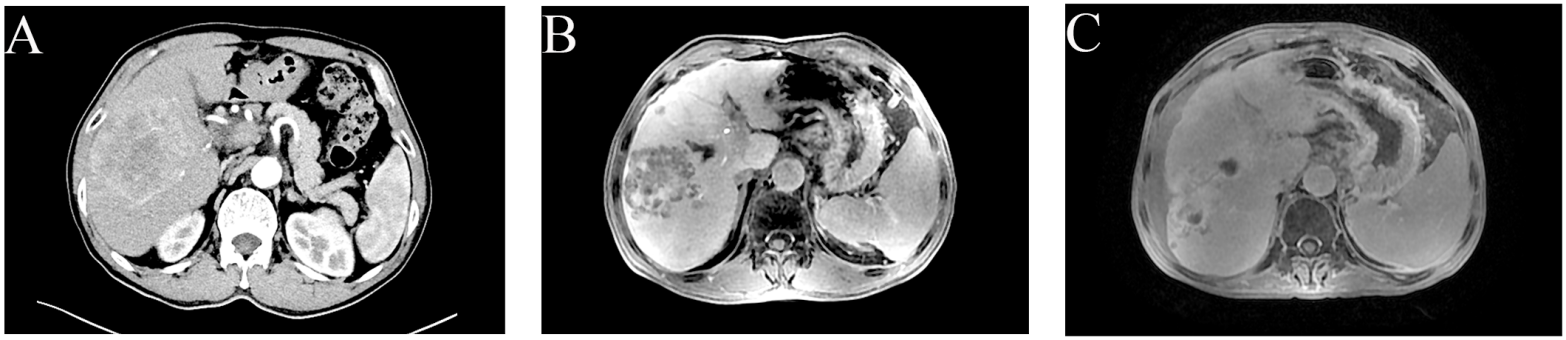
Clinical images of hepatocellular carcinoma in a 64-year-old man. (A) Advanced CT imaging has detected a significant mass in the right hepatic lobe, spanning multiple segments with dimensions of 112 mm x 77 mm x 83 mm. (B) Advanced CT imaging has identified a tumor in the right lobe of the liver with dimensions of 75 mm by 67 mm by 67 mm. Following completion of TACE, the efficacy of the treatment is PR in accordance mRECIST. (C) MRI revealed the largest hepatic tumor measuring approximately 69 mm x 63 mm x 67 mm. The efficacy of the radiotherapy has been evaluated as PR.
